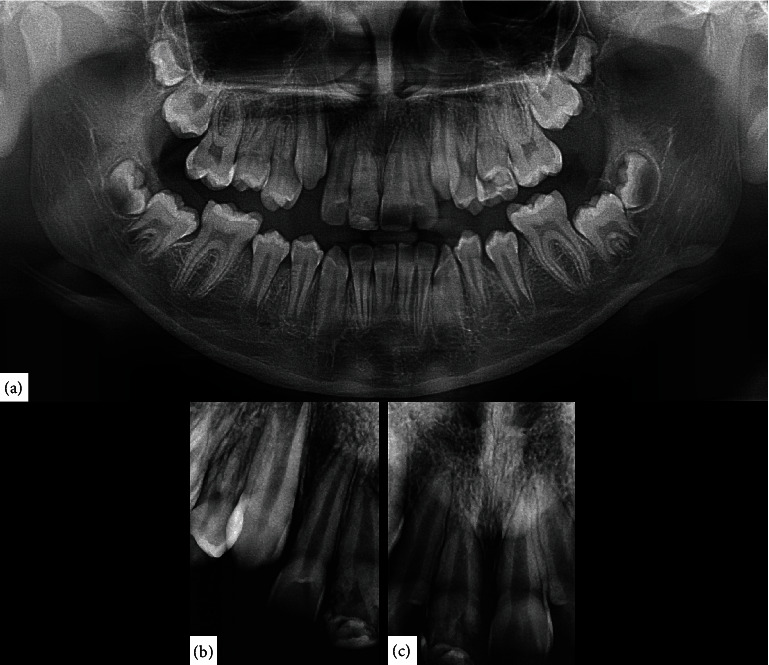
Radiological images. (a) Panoramic X-ray, showing the bone integrity at the level of the upper right central incisor. (b) Periapical radiograph, showing a horizontal coronary fracture of the upper right central incisor can be seen, affecting only enamel and dentin. (c) Periapical radiograph. It can be observed that the length of the tooth is apparently shorter compared with its contralateral counterpart; in addition, the apical region is irregular; however, its formation is complete.
